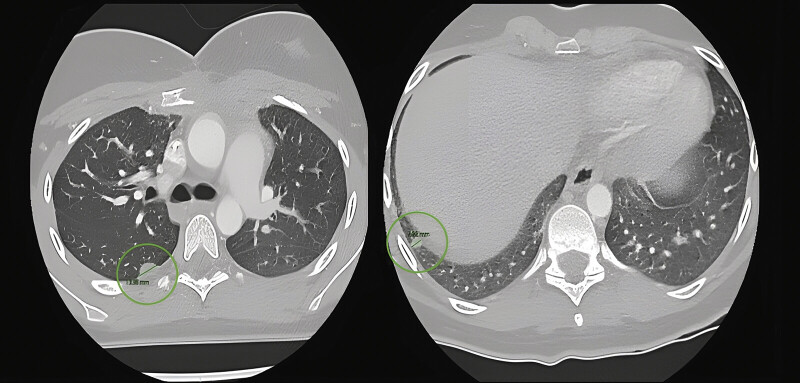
Preoperative CT scan showing metastatic nodules in segments 6 and 9.
